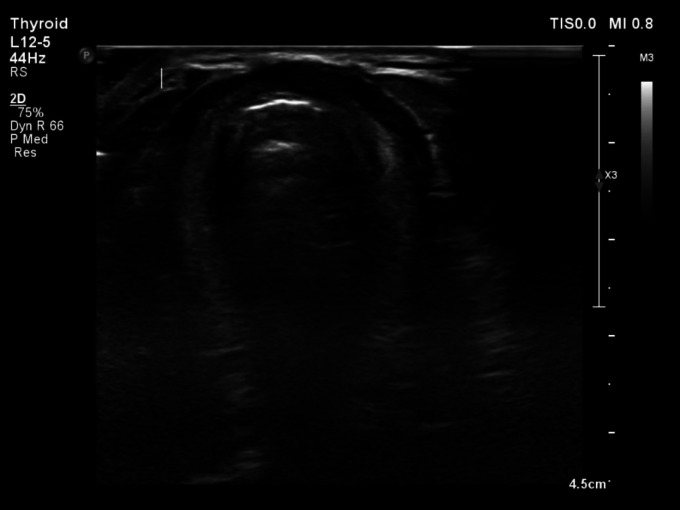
Ultrasound image of the neck demonstrating absent thyroid gland in its usual location
